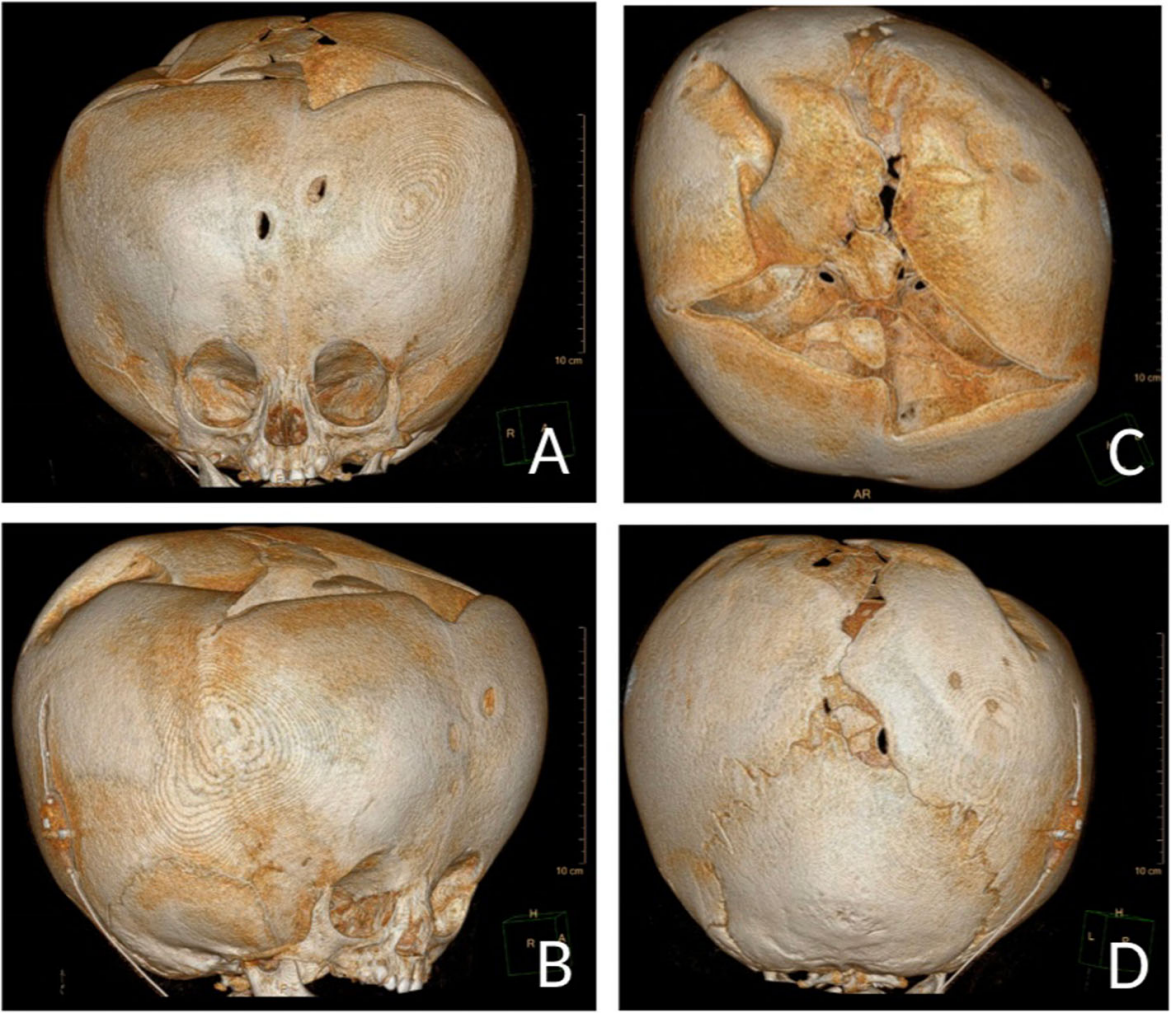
a-d 3D CT reconstruction of patient’s cranium and face after ventriculoperitoneal shunt placement
